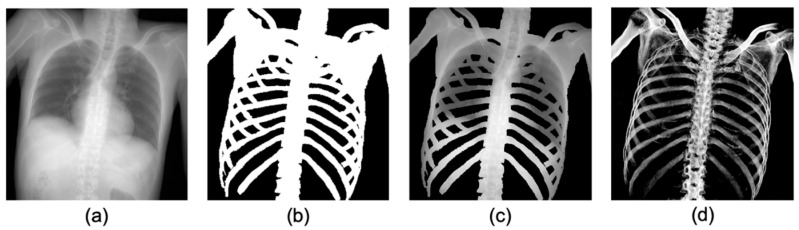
Comparison of datasets for bone extraction between diagnostic and forensic field. (a) An example of a radiograph-like image, (b) label image in diagnostic field: binary map of bone, (c) the corresponding parts for segmentation, including bone area according to label image in diagnostic field, and (d) gray-scaled bone image without soft tissues.
